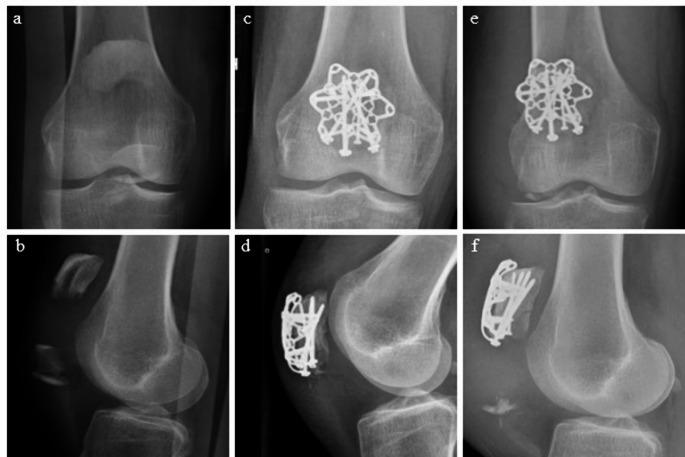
Anterior-posterior and lateral Injury (a & b), post operation (c & d) and fixation failure (e & f) radiographs of the patient who suffered distal pole fixation failure
